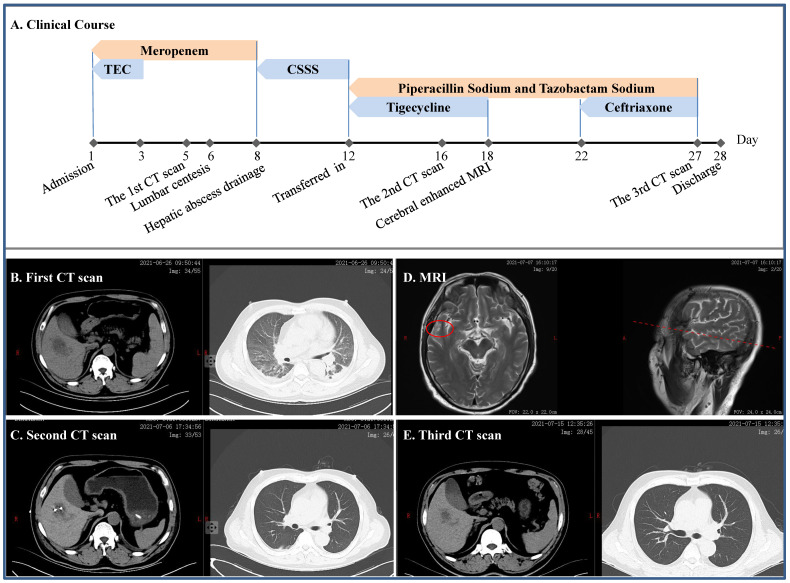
Clinical course and imaging findings in the patient with cKp infection. A: Major therapeutic procedures during the patient's hospital stay. B: The first CT scan after admission showing pulmonary infection and hepatic abscess. C: The second CT scan after the patient was transferred in showing gross normal pulmonary field and drained hepatic abscess. D: Contrast-enhanced MRI showing lesion on the right temporal lobe. E: CT scan prior to discharge. TEC and CSSS: teicoplanin and cefoperazone sodium and sulbactam sodium, respectively.
